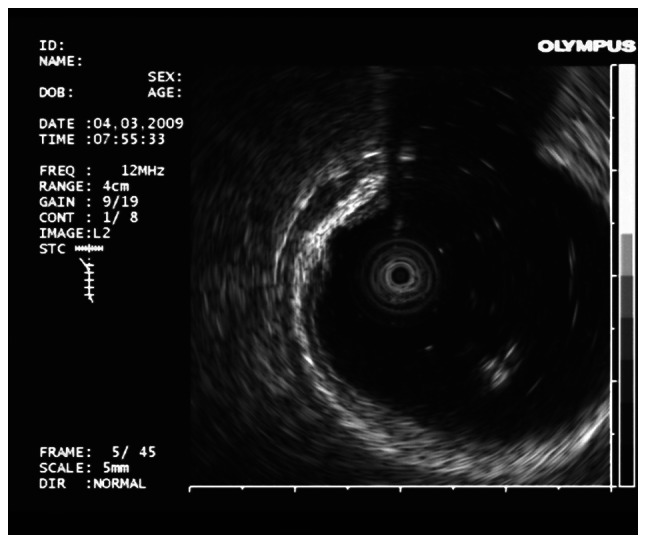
Ultrasonographic examinations showed no local carcinoma after excision.
